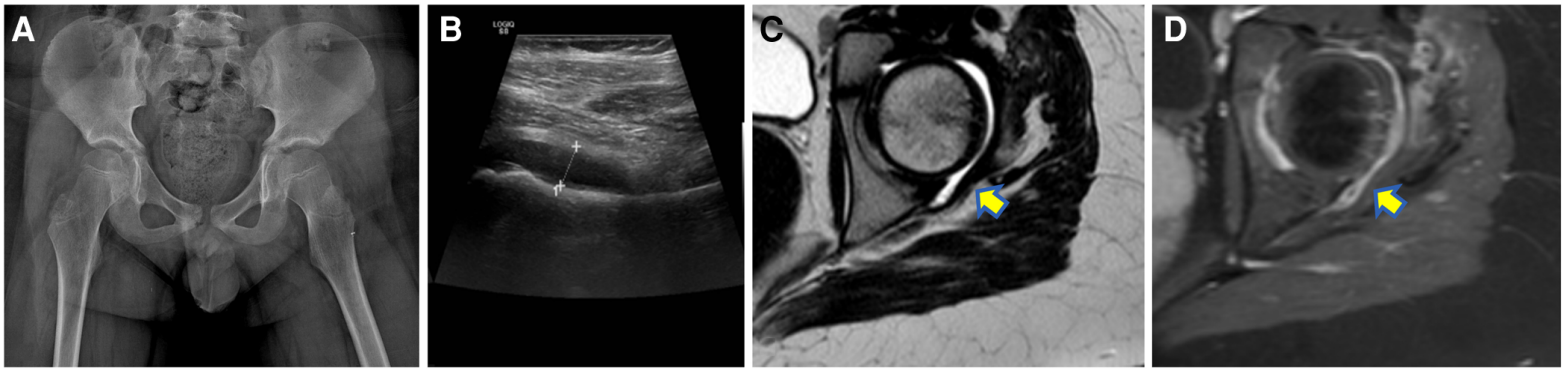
A 10-year-old boy with left hip pain 2 weeks after a mild respiratory COVID-19 infection with negative radiographs (A) but joint effusion at ultrasound (B) then confirmed at the MR [yellow arrow on the axial T2W turbo spin echo sequence in (C)] which also demonstrated synovial enhancement after contrast medium injection [yellow arrow in (D)].
